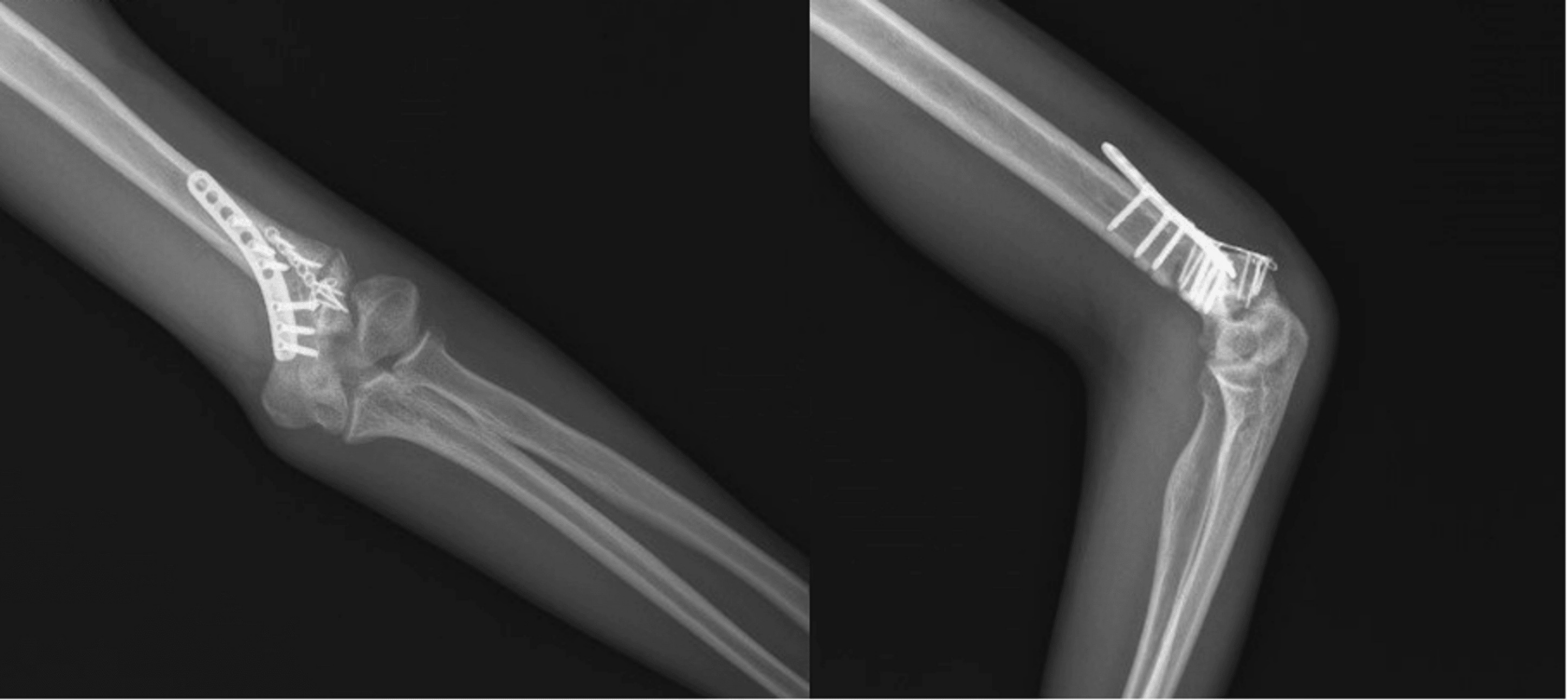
Radiographic images of the patient taken on the first postoperative day
